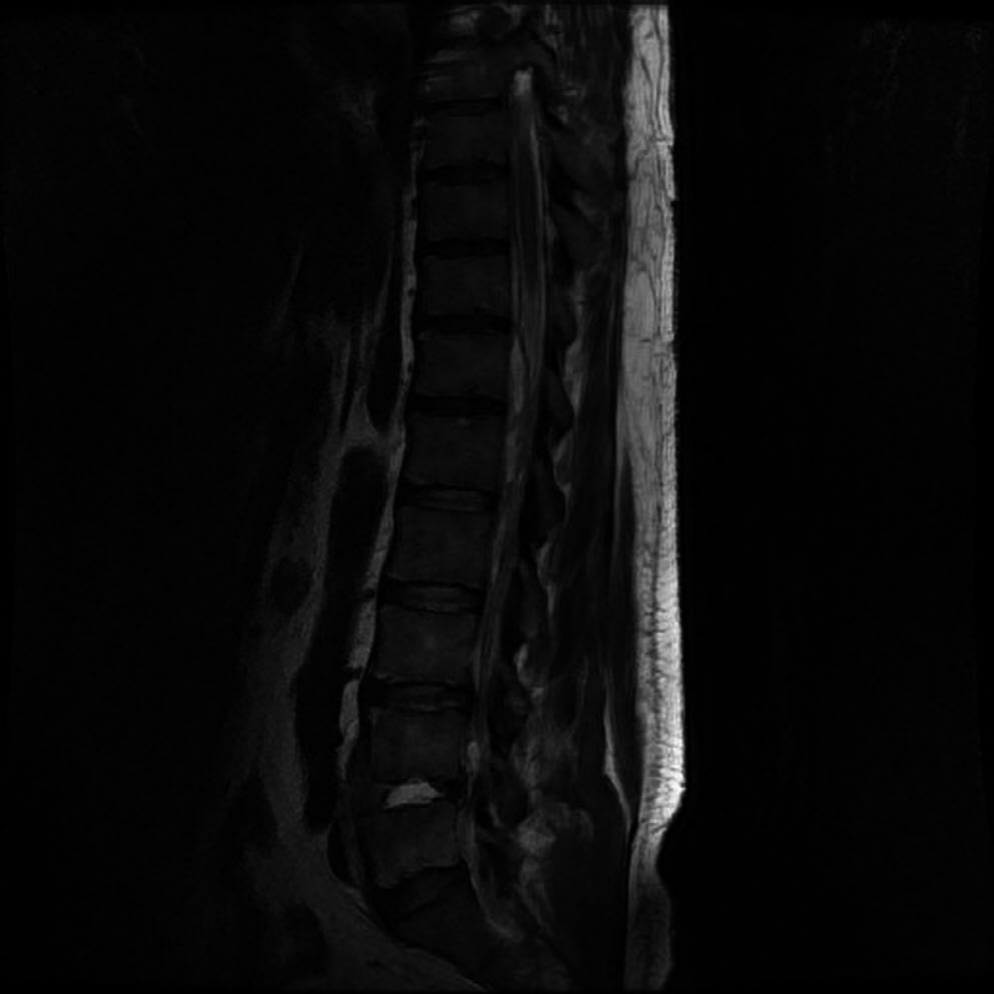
Lumbar magnetic resonance imaging on the second day of hospitalization. The image shows an extensive epidural abscess with evidence of discitis in the L5/S1 region
